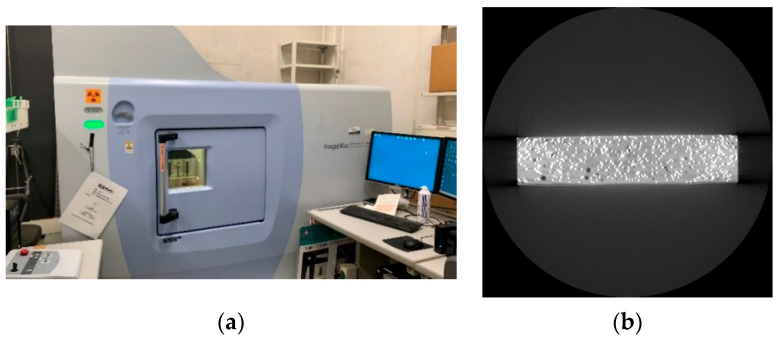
CT scan process. (a) Shimadzu inspeXio SMX-225CT FPD HR Plus scanner. (b) CT image of transversal section.
